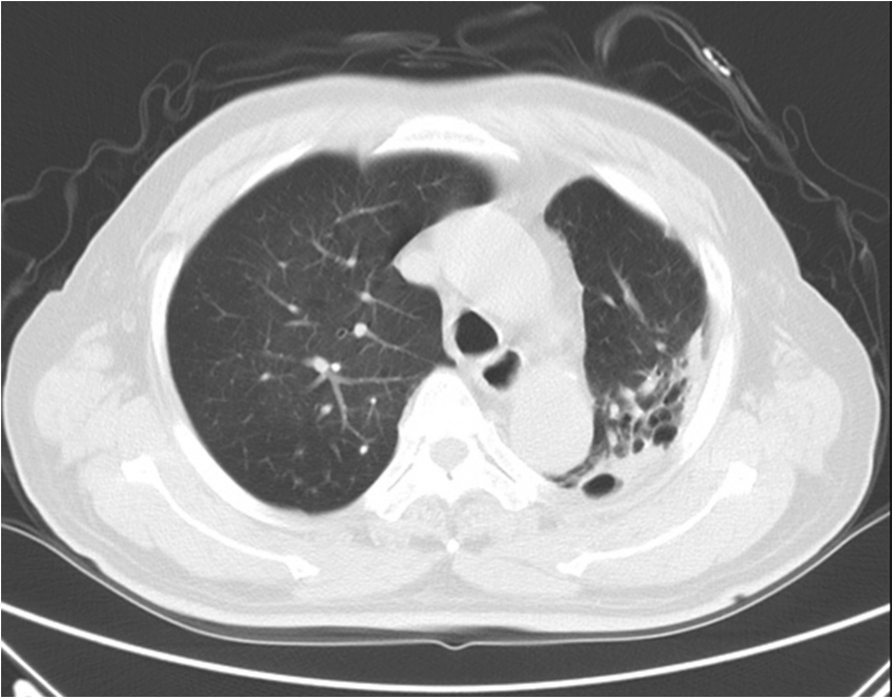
Thin section CT of an 86 –year-old male with NTM (
